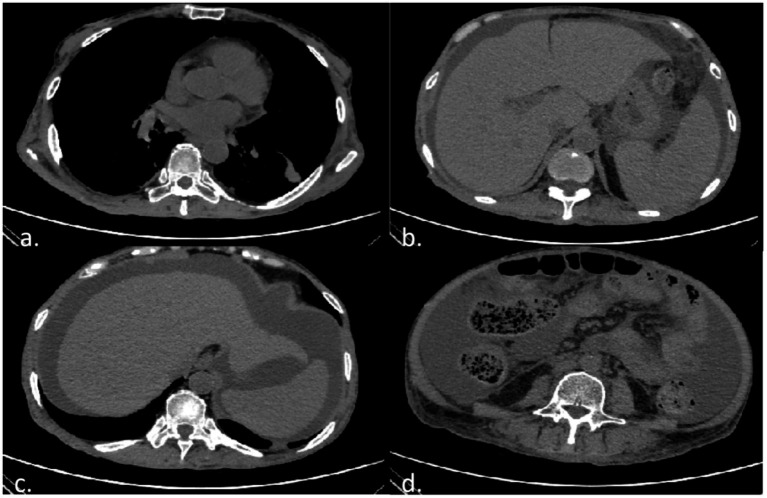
CT imaging of the chest, abdomen, and pelvis demonstrating multisystem manifestations. (a) Chest CT demonstrates pericardial effusion. (b) Abdominal CT shows an irregular, wavy liver contour and splenomegaly. (c) Abdominal CT reveals free intraperitoneal fluid accumulation. (d) Pelvic CT shows thickened, edematous intestinal walls and subcutaneous edema.
